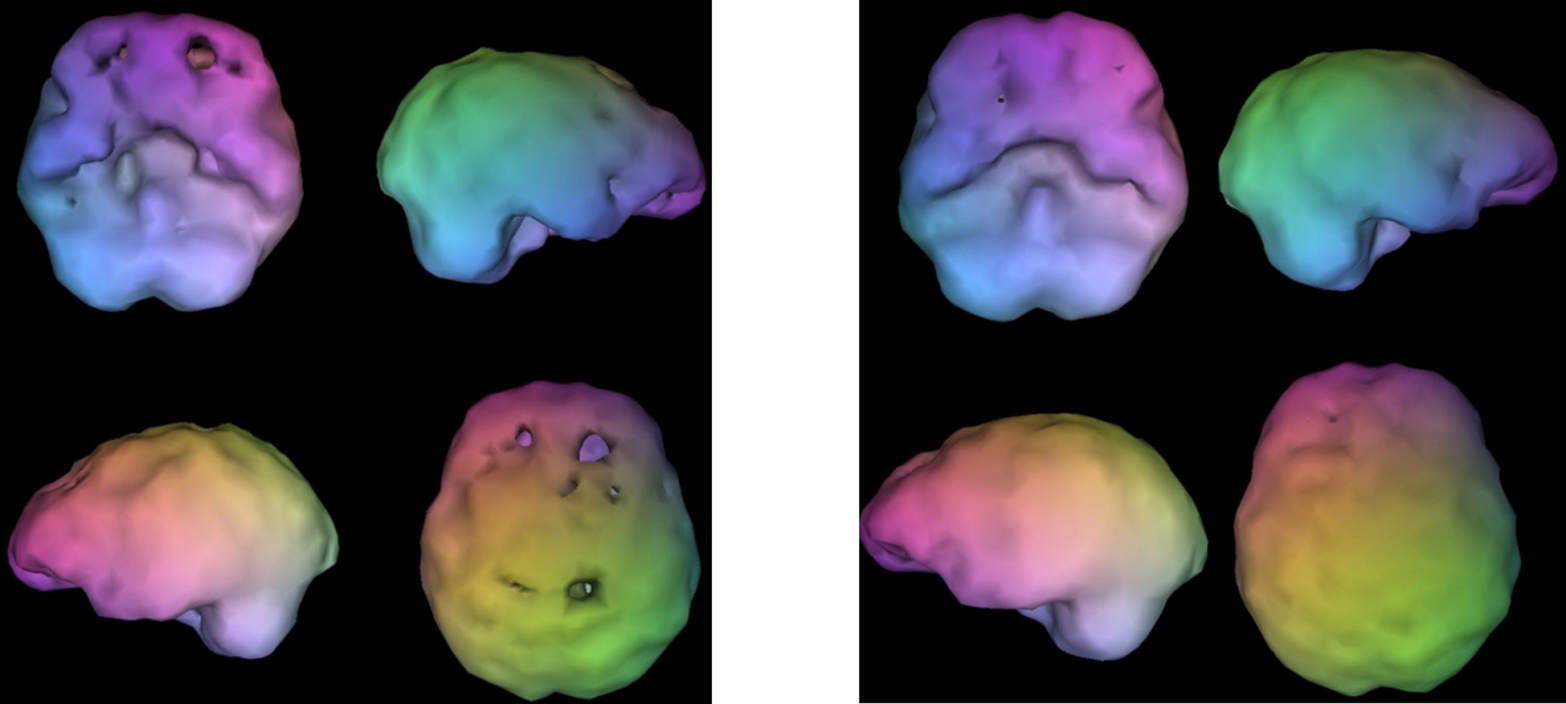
SPECT surface images without medication (L) and on mixed amphetamine salts (R). Without medication, SPECT images (L) reveal overall decreased prefrontal perfusion and post-treatment images (R) show improved prefrontal perfusion.
